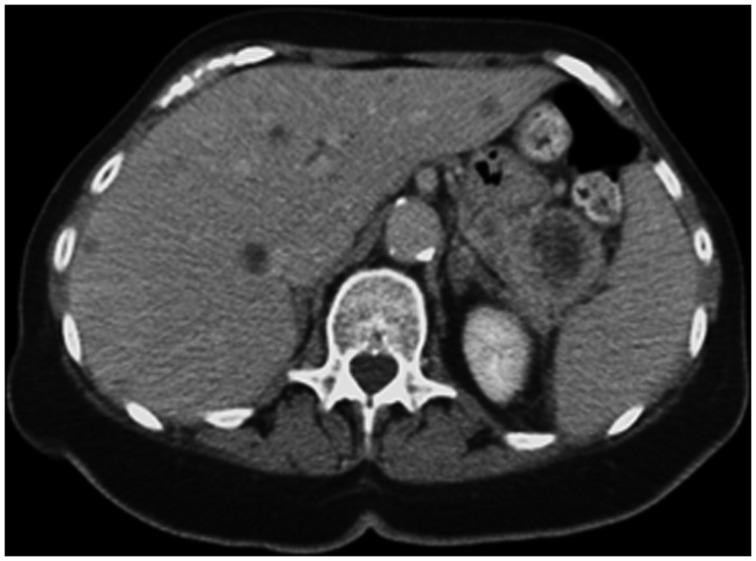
Abdominal computed tomography reveals a 5 × 3.7 cm pancreatic-tail mass with central necrosis and multiple lesions throughout the liver, with central low attenuation.
